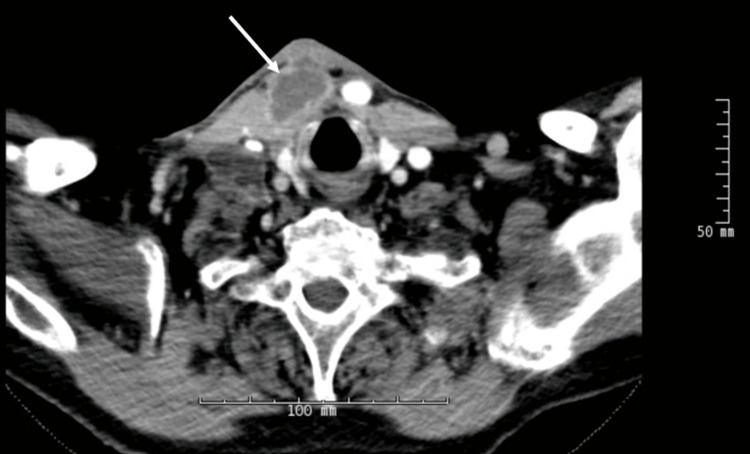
Soft tissue neck CT with IV contrast
